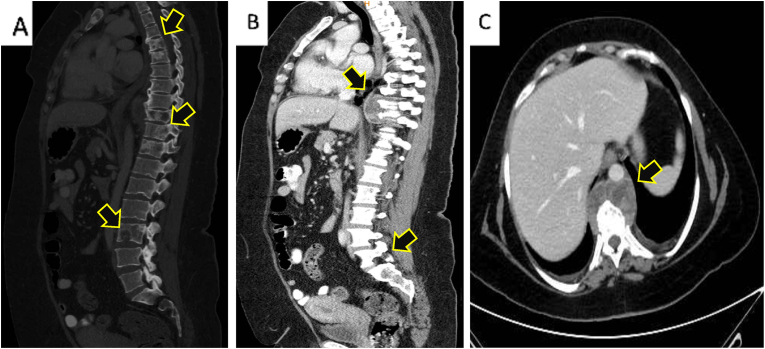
(A): is a Computed Tomography (CT) scan with bone window, (B): is a CT scan with soft tissue window, and (C): is a CT scan with coronal view demonstrating para-spinal soft tissue lesion at the level of T8-T9, (Arrows define the significant findings).
